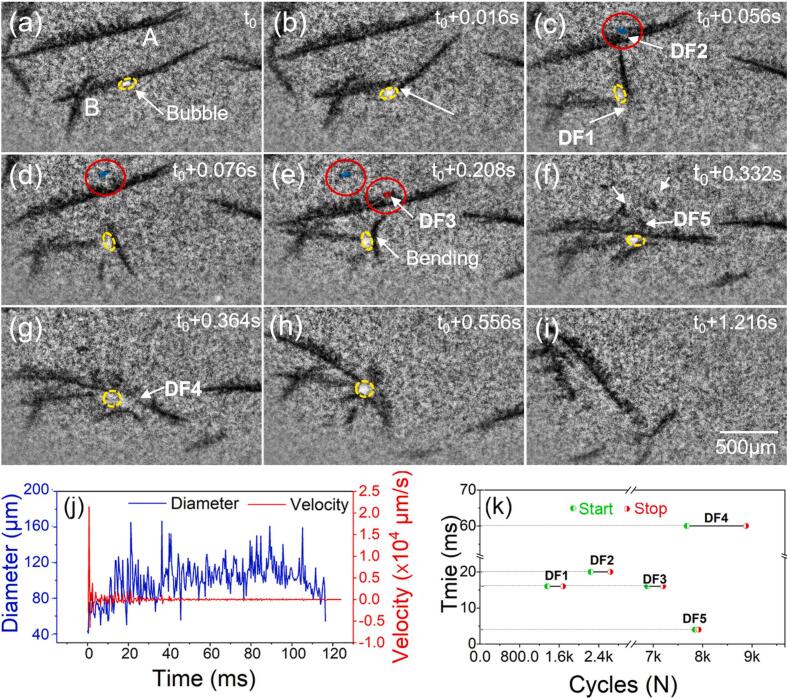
(a-i) A series of images that show clearly the oscillating bubbles induced dendrite fragmentation events. (j) The diameter and wall velocity of the marked bubble. (k) the time to complete the fragmentation of the marked dendrites. Experimental conditions: 30 W ultrasound, 1137.5 K/m thermal gradient, 250 fps (see Video 5).
